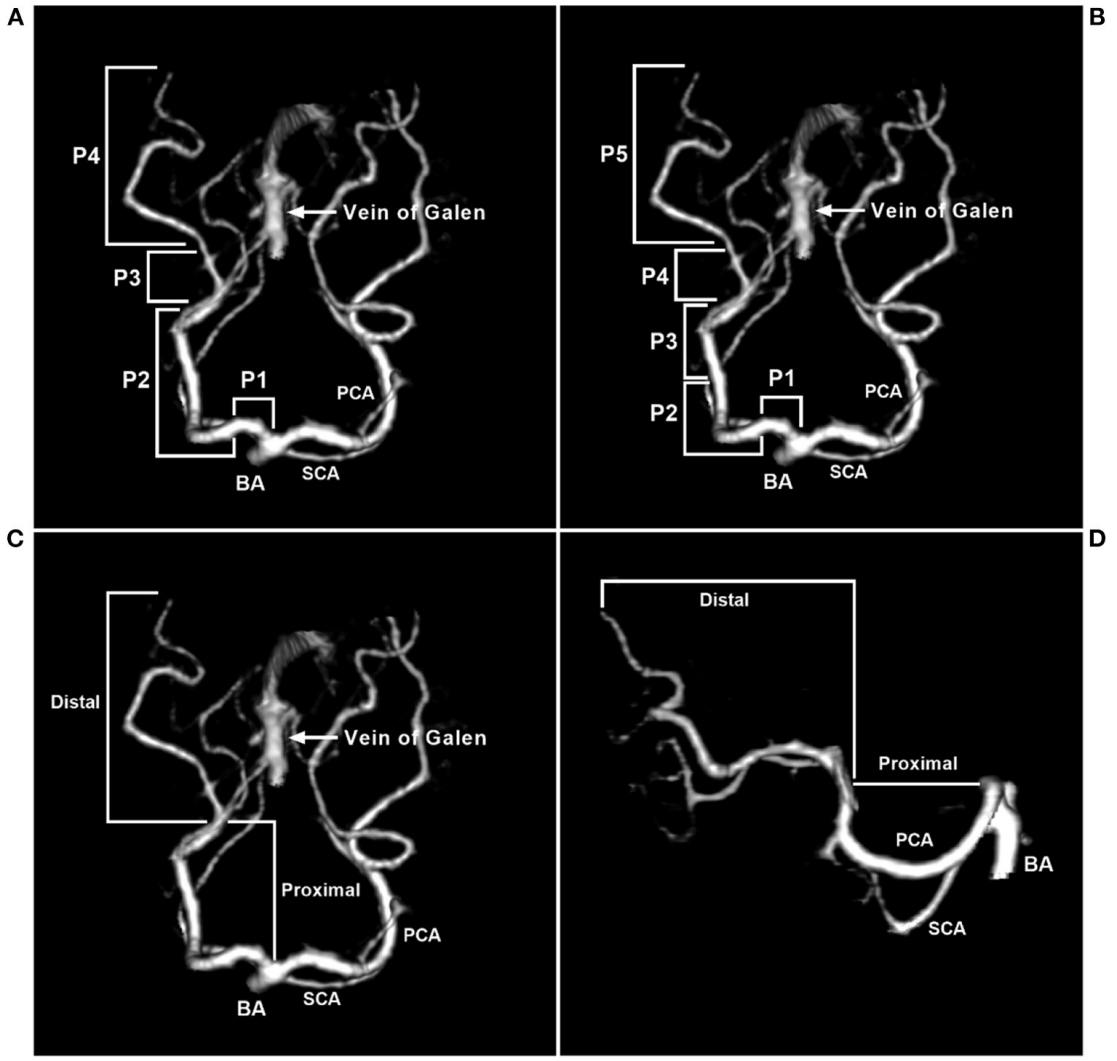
Segmentation of the PCA. (A) CTA of the superior-inferior view showing that the PCA was divided into four segments: P1 (precommunicating segment), P2 (ambient segment), P3 (quadrigeminal segment), and P4 (calcarine segment). P2 was further divided into anterior (P2A) and posterior (P2P) parts. (B) CTA of the superior-inferior view showing that the PCA was divided into five segments. (C,D) CTA of the superior-inferior view (C) and the lateral view (D) showing that the PCA can be divided into proximal and distal segments. BA, basilar artery; CTA, computed tomography angiography; PCA, posterior cerebellar artery; SCA, superior cerebellar artery.
